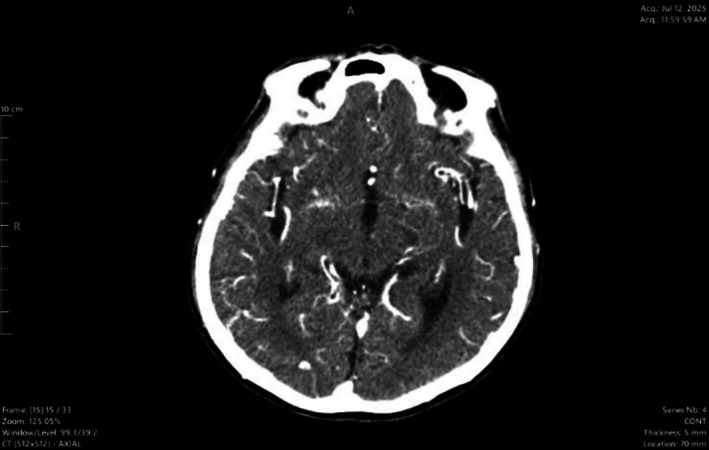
Initial CT Brain done at Emergency—showing Leptomeningeal enhancement.
